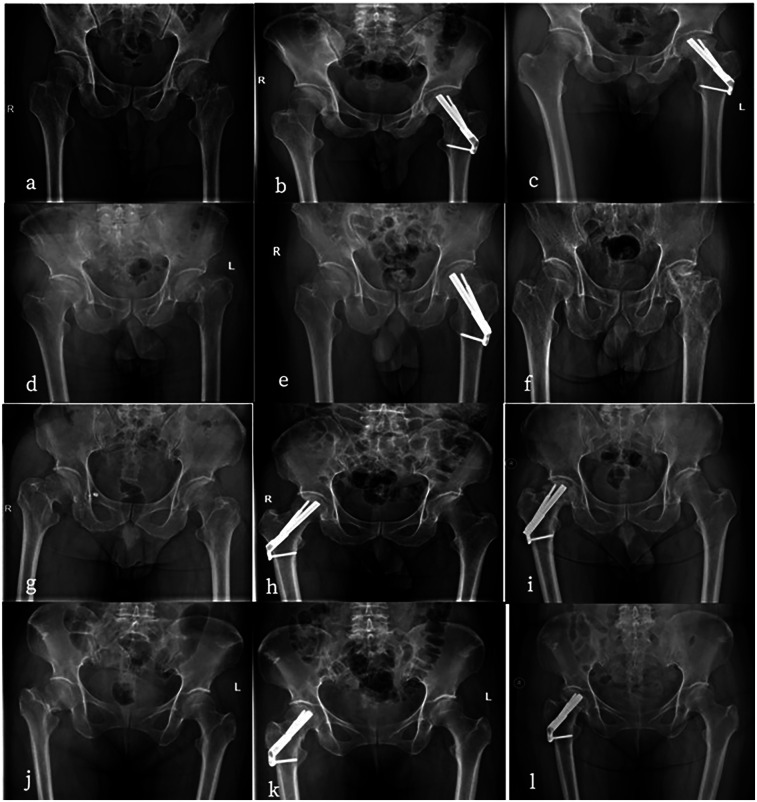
(a–c) Are all 56-year-old males. a has a preoperative anteroposterior hip radiograph showing a left femoral neck fracture (Garden IV type) with severe displacement. (b) Is a postoperative anteroposterior radiograph after FNS surgery. (c) developed femoral head necrosis two years after surgery. (d–f) Are all 35-year-old males.d has a preoperative anteroposterior radiograph of the hip showing a left femoral neck fracture (Garden IV type). (e) Is a postoperative anteroposterior radiograph after FNS surgery. (f) developed femoral head necrosis two years after the removal of the FNS internal fixation. (g–i) Are all 48-year-old females. (g's) preoperative anteroposterior hip radiograph shows a left femoral neck fracture (Garden IV type). (h) Is in a near-anatomic reduction position postoperatively. (i) Began weight-bearing two weeks after surgery and developed femoral head necrosis six months after follow-up. (j–l) Are all 36-year-old females. (j's) preoperative anteroposterior radiograph of the hip shows a right femoral neck fracture (Garden IV type). (k) Is a postoperative anteroposterior radiograph after FNS surgery with poor reduction quality. (l) Developed femoral head necrosis one year after surgery.
